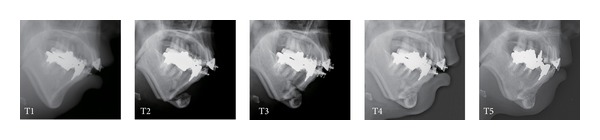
Lateral cephalograms at each step in Case 1. T1: immediately before genioplasty; T2: immediately after genioplasty; T3: immediately after periapical segmental alveolar osteotomy; T4: immediately before two-jaw surgery; T5: at debonding.
